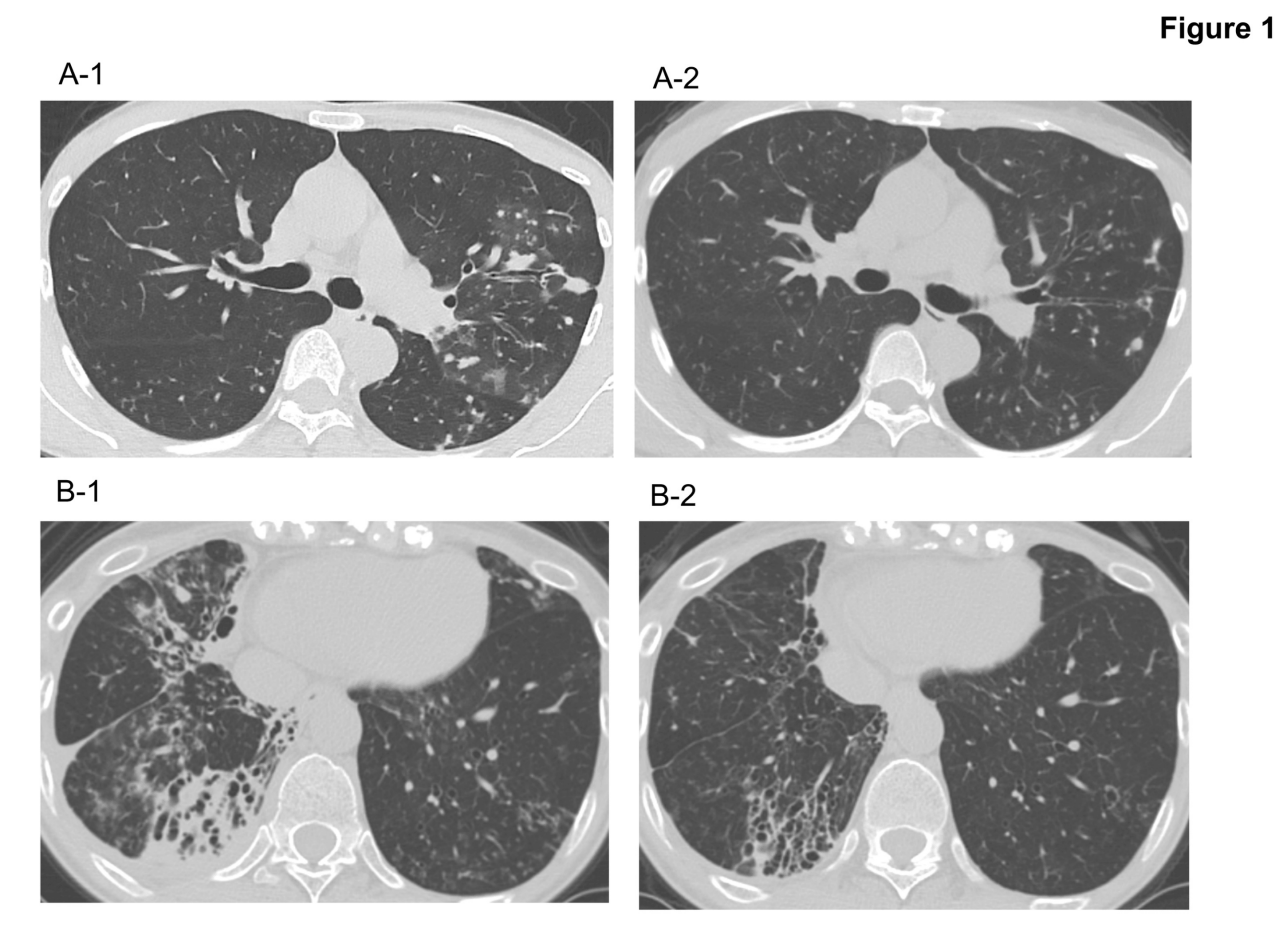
Chest CT before and after administration of STFX and ALIS in Cases 1 (responder) and 2 (responder) of CAM-resistant pulmonary MAC disease (A-1) Chest CT before STFX+ALIS in case 1. (A-2) Chest CT after STFX+ALIS in case 1. (B-1) Chest CT before STFX+ALIS in case 2. (B-2) Chest CT after STFX+ALIS in case 2. Abbreviations STFX: sitafloxacin, ALIS: amikacin liposome inhalation suspension, CAM: clarithromycin, MAC: *Mycobacterium avium* complex
